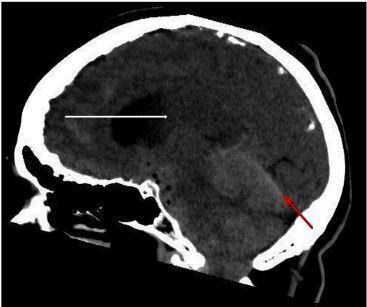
Computed tomography of head showing subdural hemorrhage (red arrow) and intraventricular hemorrhage (white arrow).
